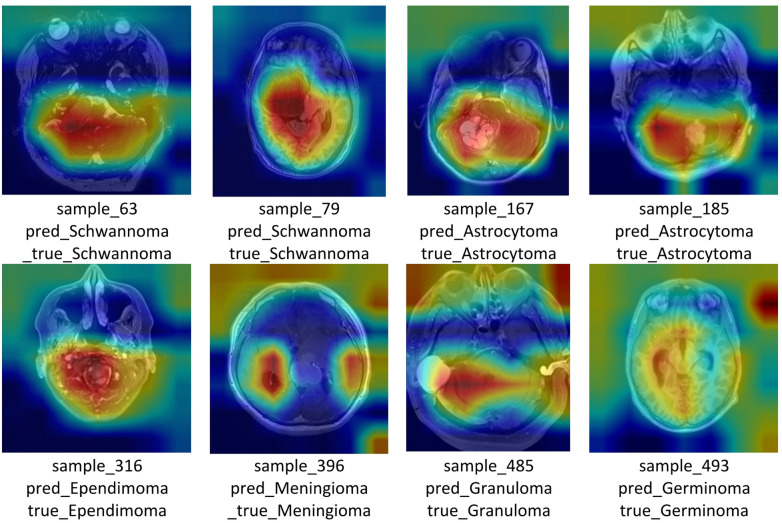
Representative Grad-CAM visualizations for correctly classified MRI brain tumor samples.
